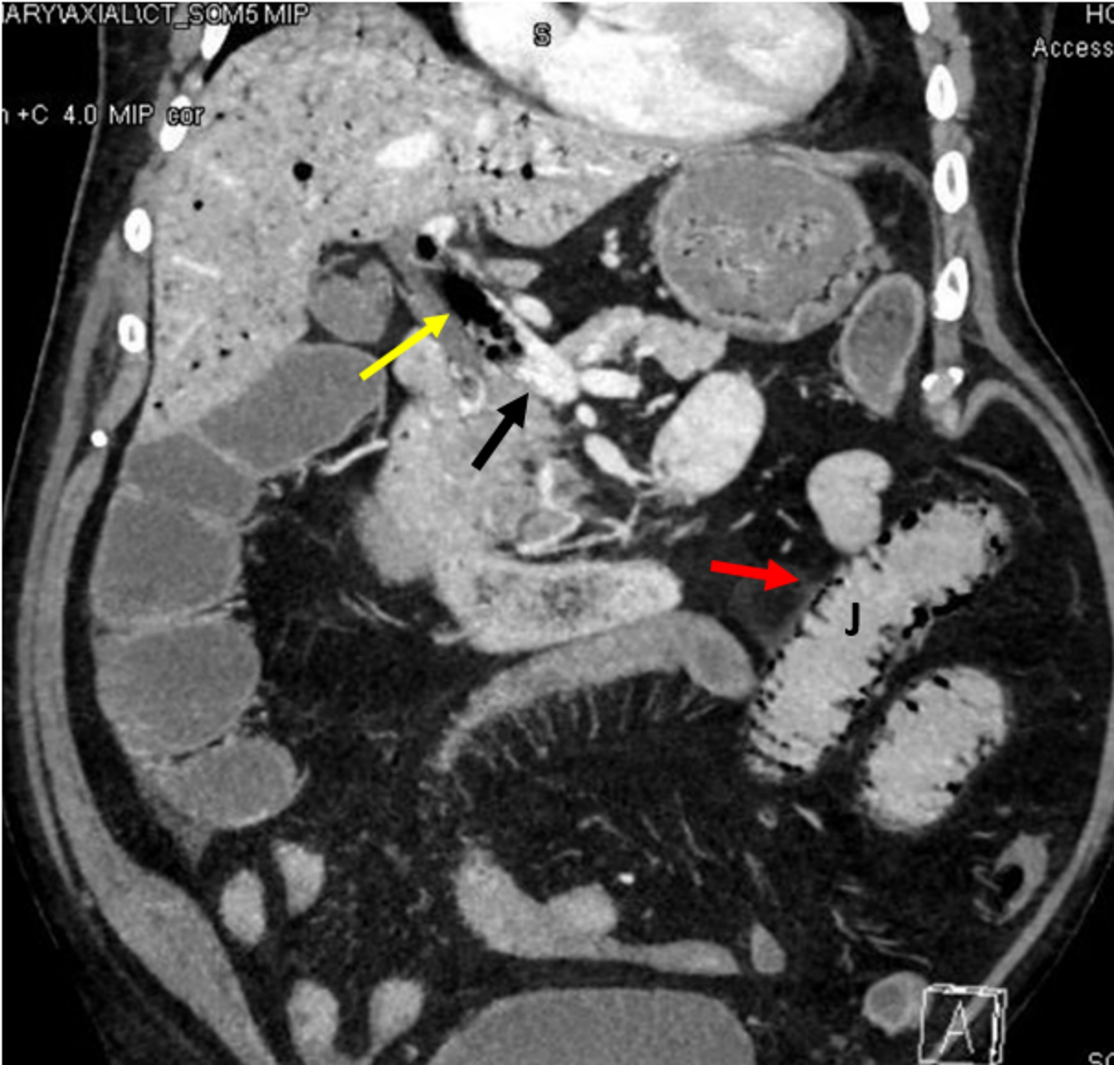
Coronal view CT scan, the black arrow denotes portal vein with gas bubbles labeled by the yellow arrow. The red arrow shows pneumatosis intestinalis in the wall of the jejunum (J).
